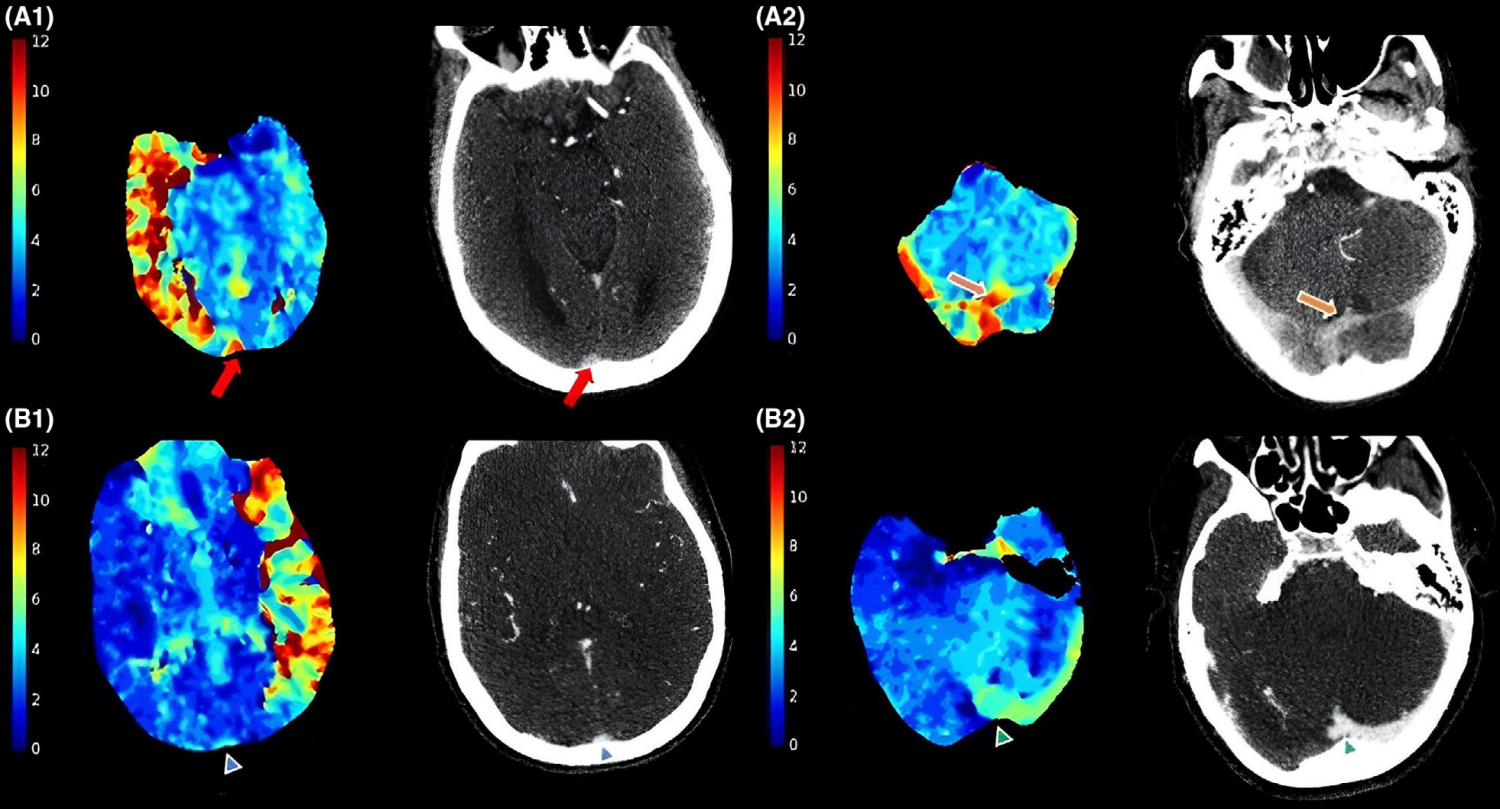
(A and B) PVT assessment in post‐processed Tmax maps (left) and corresponding axial CTA (right) views of two patients. (A1 and A2) A 64‐year‐old patient presented with a right internal carotid artery occlusion and admission NIHSS score of 18, and then discharged with NIHSS percent change of 61% at discharge. This patient was PVT+ as Tmax ≥10 s was observed in the posterior superior sagittal sinus at the level of the occipital horns (A1, red arrow) and at the torcula (A2, orange arrow). (B1 and B2) A 64‐year‐old patient presented with a left M1 middle cerebral artery occlusion and admission NIHSS score of 8, and then discharged with NIHSS percent change of 100%. This patient was PVT− as Tmax ≥10 s was not present either in the posterior superior sagittal sinus (B1, blue arrowhead) or at the torcula (B2, green arrowhead). CTA, CT angiography; NIHSS, National Institutes of Health Stroke Scale; PVT, prolonged venous transit; Tmax, time to maximum.
